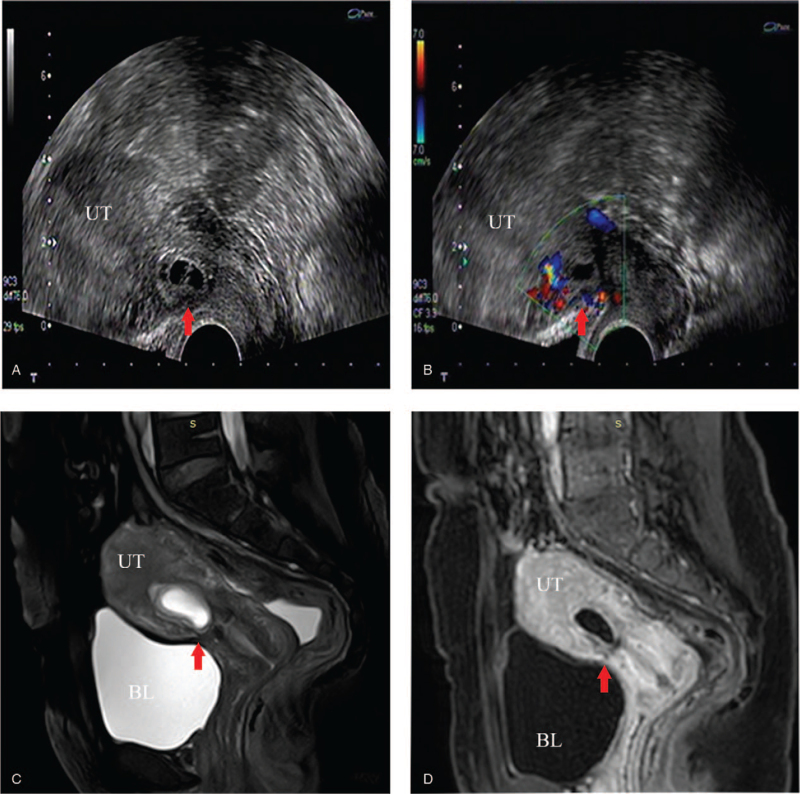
US and MRI images of CSP (from the author's institution). Transvaginal 2-dimensional grayscale (A) and color Doppler (B) ultrasound. Sagittal T2-weighted image (C) and sagittal T1-weighted image (D) of MRI. Gestational sac implantation site (red arrows). BL = bladder, CSP = cesarean scar pregnancy, MRI = magnetic resonance imaging, US = ultrasonography, UT = uterus.
